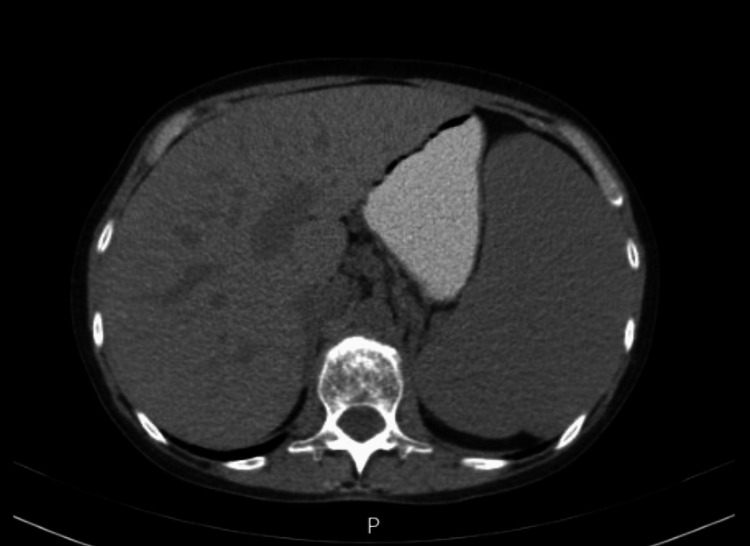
Abdominal CT scan demonstrating hepatosplenomegaly Diffuse enlargement of the liver with caudate lobe hypertrophy and homogeneous splenomegaly are observed, without evidence of abdominal lymphadenopathy.
